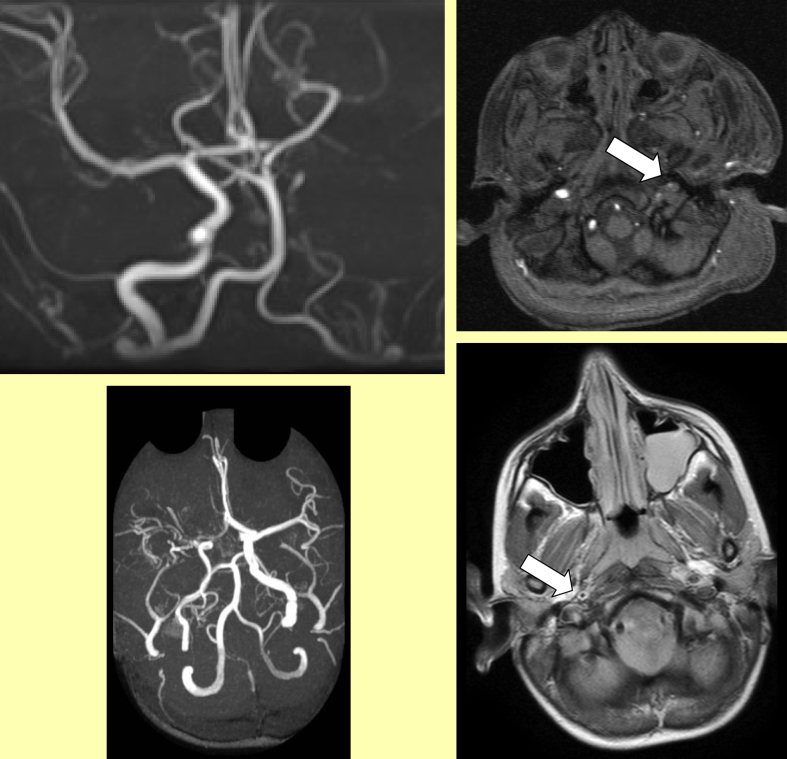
Figure 5: Left: Asymmetry of flow in the cerebral vessels in 2 children with stroke. Right: Fat-saturated T1 MRI of the neck showing carotid occlusion (Top) after trauma from a pencil after a 2 year old fell with it in his mouth (see Figure 11) and the blood in the vessel wall in a child with an apparently spontaneous dissection.
